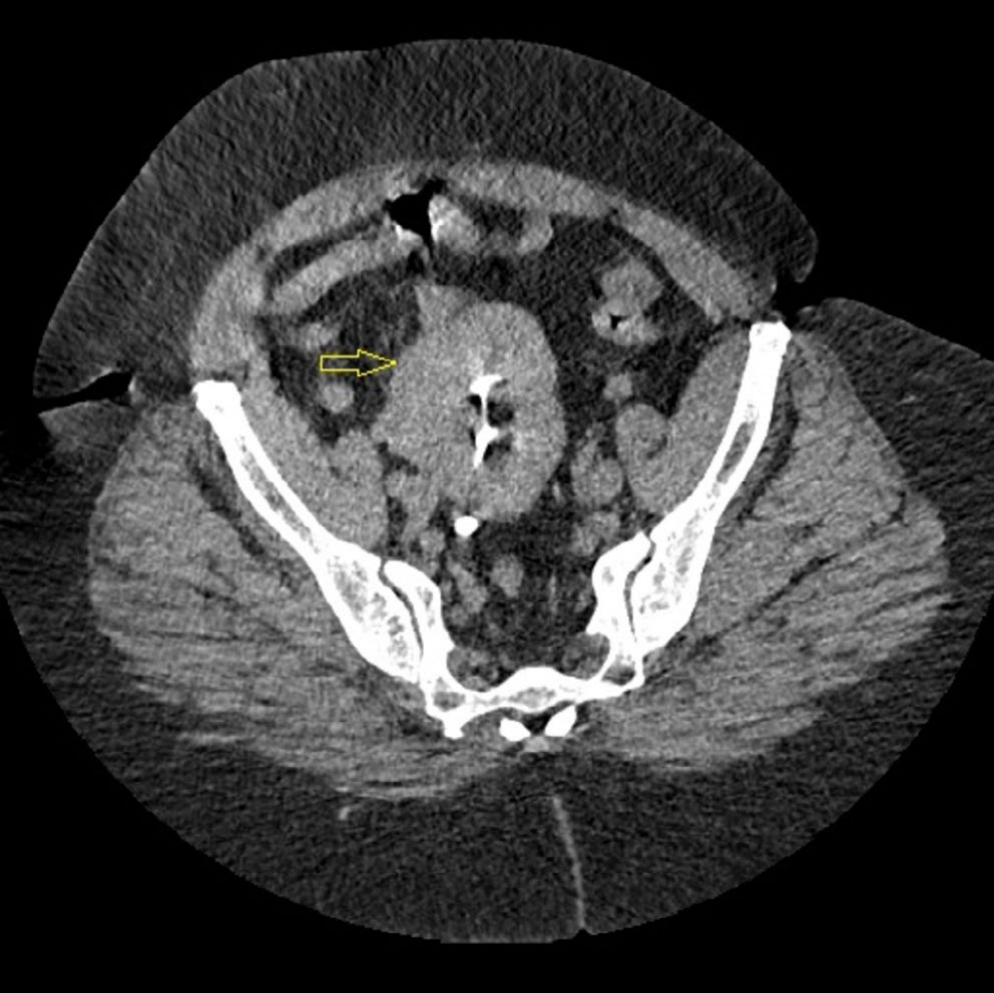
Axial view, contrast‐enhanced CT scan. The image shows the ectopic pelvic kidney (yellow arrow) located in the pelvis, between the two iliac crests.
